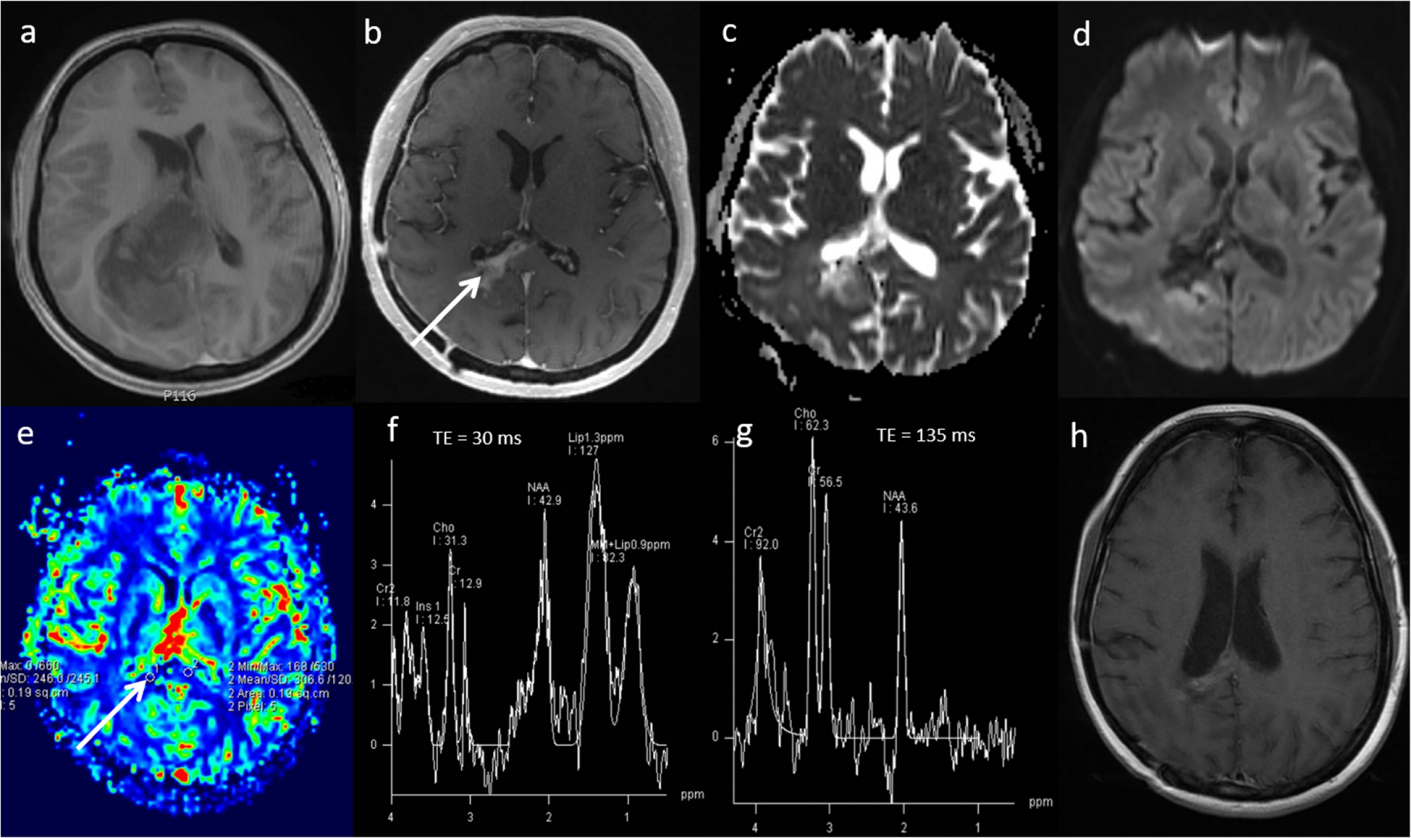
Glioblastoma—pseudoprogression. a Pre-operative T1-weighted image shows a right deep parietal region glioblastoma. b Conventional post-contrast T1-weighted image approximately 4 weeks after chemoradiotherapy treatment demonstrates a significant increase in the contrast-enhancing area (arrow). Multiparametric MRI at this timepoint demonstrates: c, d areas of high ADC (1186 × 10^−6^ mm^2^ s^−1^), (e) a low rCBV ratio (1.4, arrow) on PWI, (f, g) a low Cho/Cr ratio (1.4), a low Cho/NAA ratio and presence of lipid and lactate on MRS. Combination of parameters suggest pseudoprogression. h Clinical follow-up and conventional post-contrast T1W sequence at six months confirms a decrease in the amount of enhancing disease, indicating pseudoprogression
